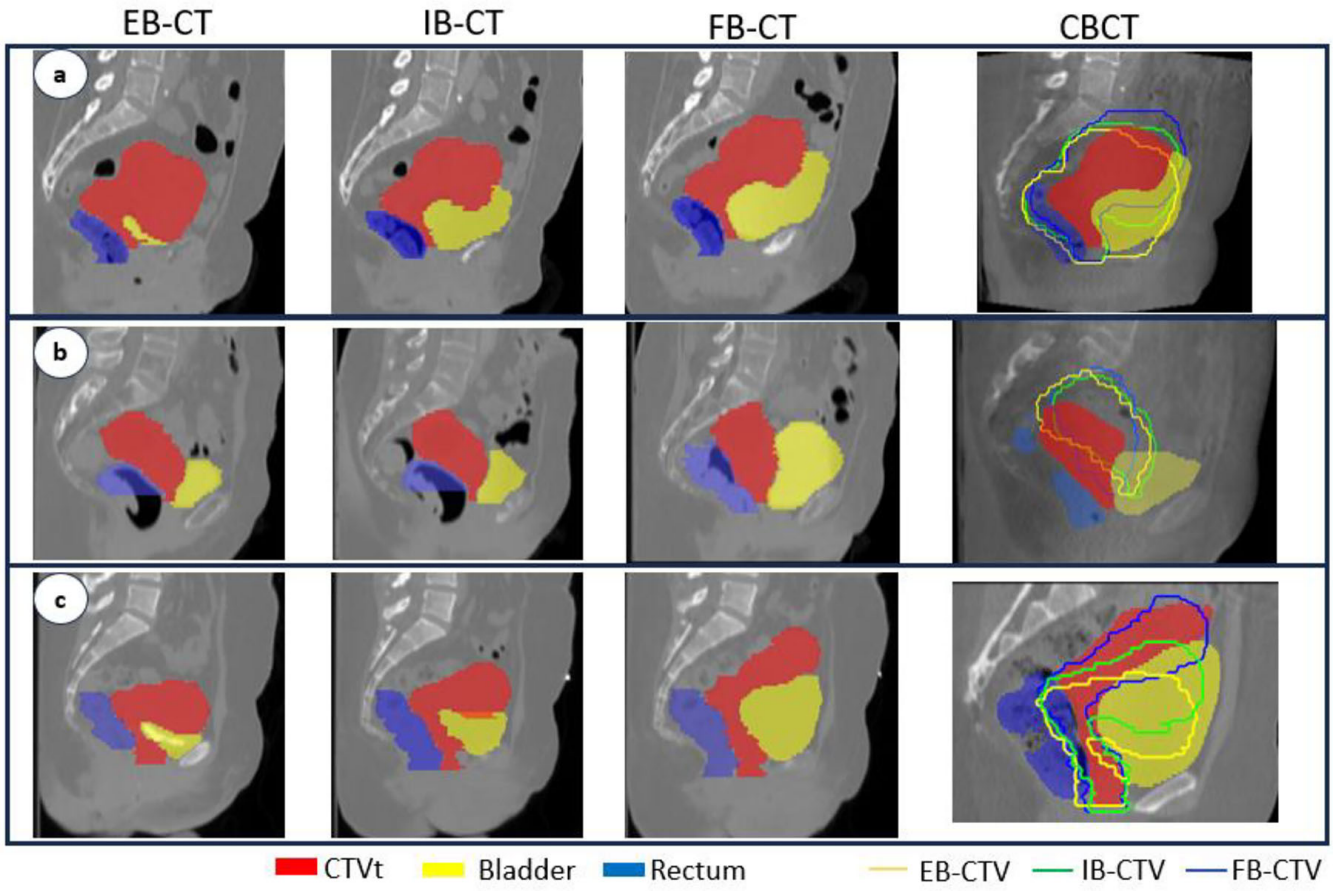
For each case: the three planning CTs (Empty bladder EB, Intermediate bladder IB and Full bladder FB) followed by the CBCT of the day with the daily CTVt (red) and CTVts from planning CT overlaid. Examples of cases: (a) Concordant PoD choice (FB plan); (b) Major discordant choice: CTVt located outside all three planning CTs (FB instead of EB); (c) suboptimal manual choices without justification (EB instead of FB).
